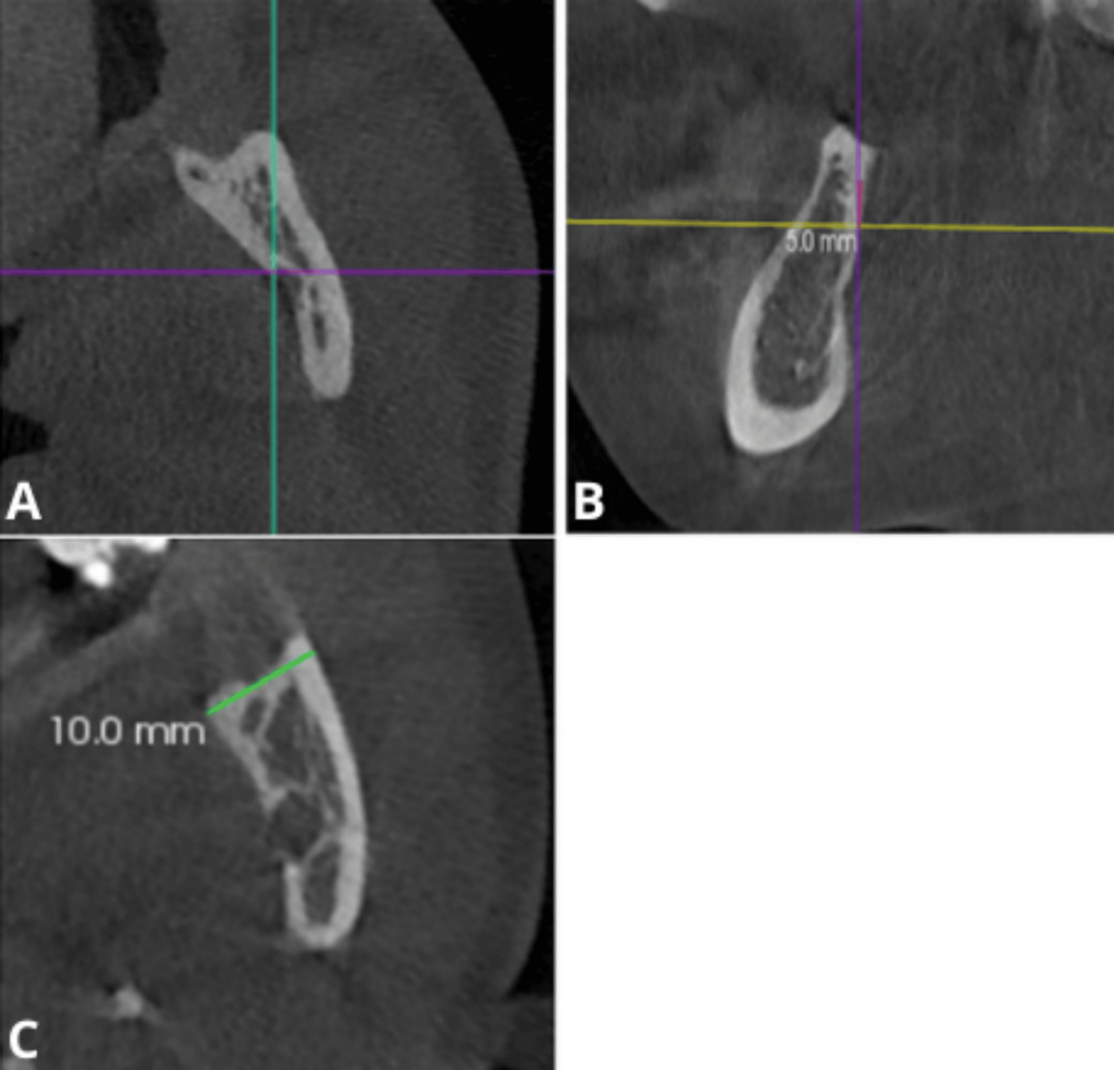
On the axial view of the cone-beam computed tomography (CBCT), the most prominent point of the lingula, considered the skeletal reference point, is identified. This point is located at the intersection of the green and purple lines (A). On the sagittal plane, a measurement of 5 mm is marked above the identified skeletal reference point to establish a new reference level (B). The axial slider is then adjusted to this level, and the maximum transverse width of the ramus is measured on the axial plane (C).
